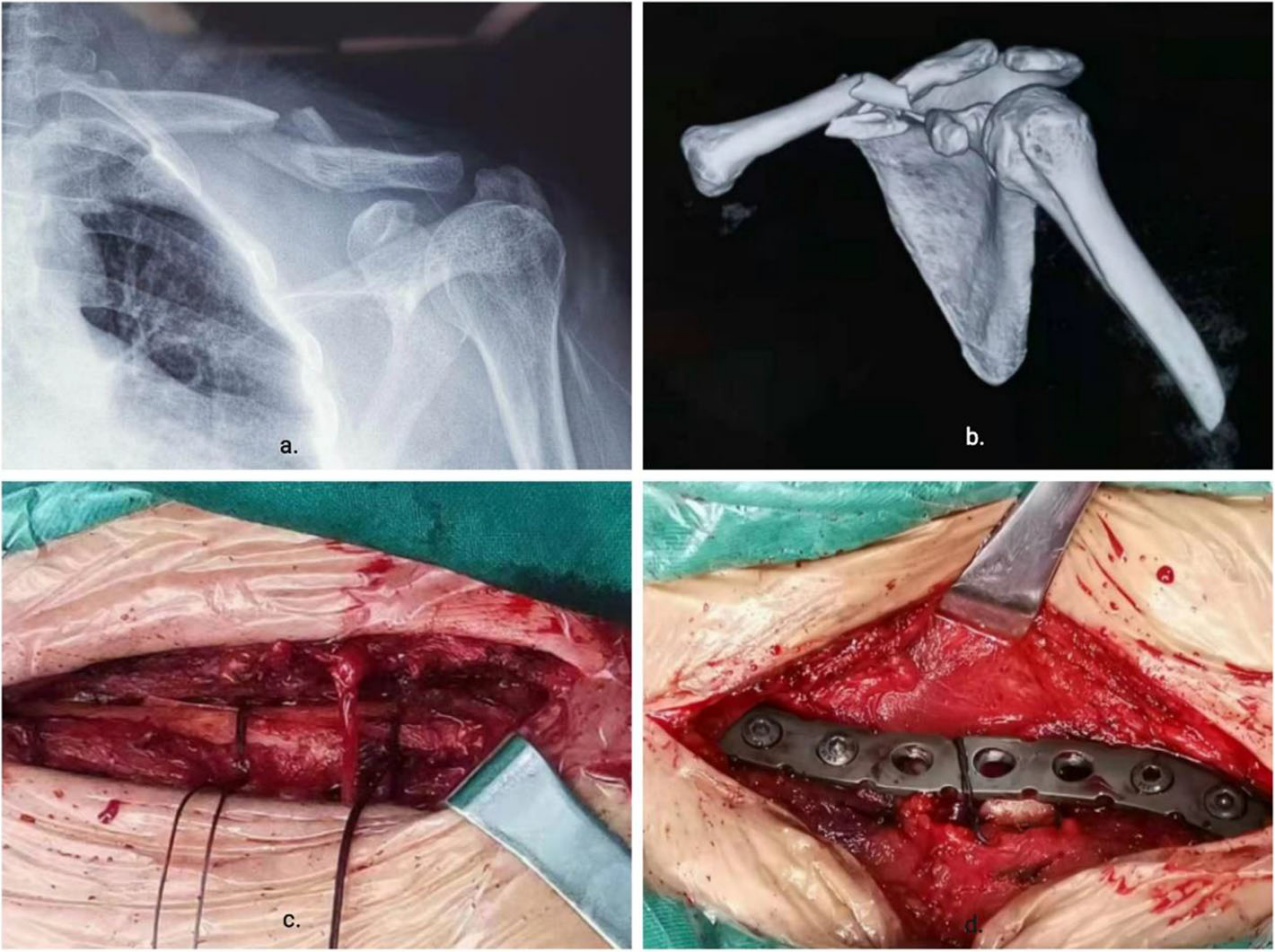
Intra-operative application of the Nice knots as an auxiliary reduction technique. a, b Pre-operative X-ray and CT indicate a displaced comminuted fracture of the clavicle. c Exposure of the main fracture line with the preservation of the rest of the periosteum and Nice knots were introduced surrounding the “Surrounding” of the fractured fragments and progressively tightened. d After proper placement of steel plates and screws, nice knot is used to strengthen the steel plates and clavicles
